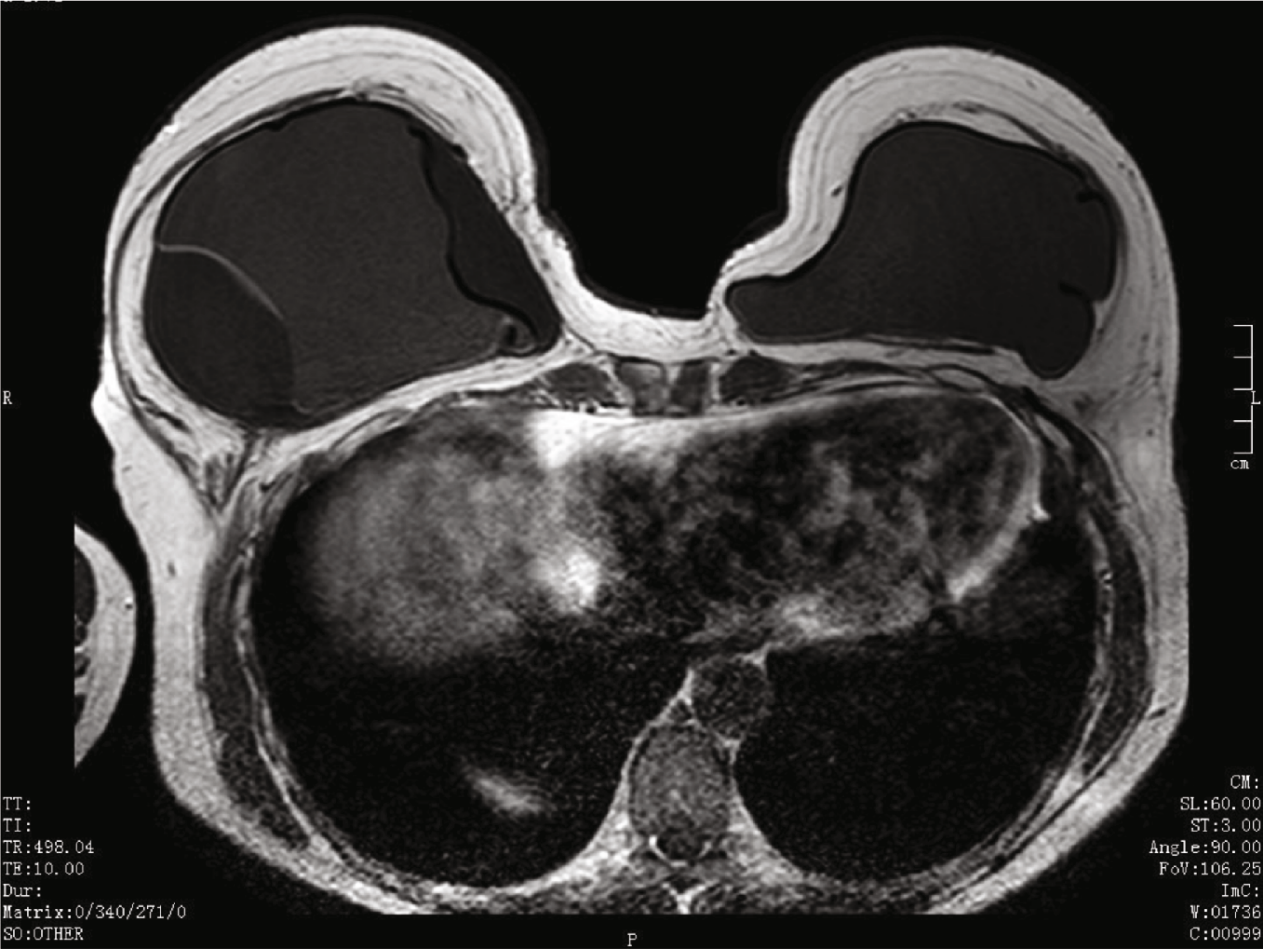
Breast MRI. Smooth circumferential thickening of the right implant fibrous capsule noted with diffuse progressive enhancement.
